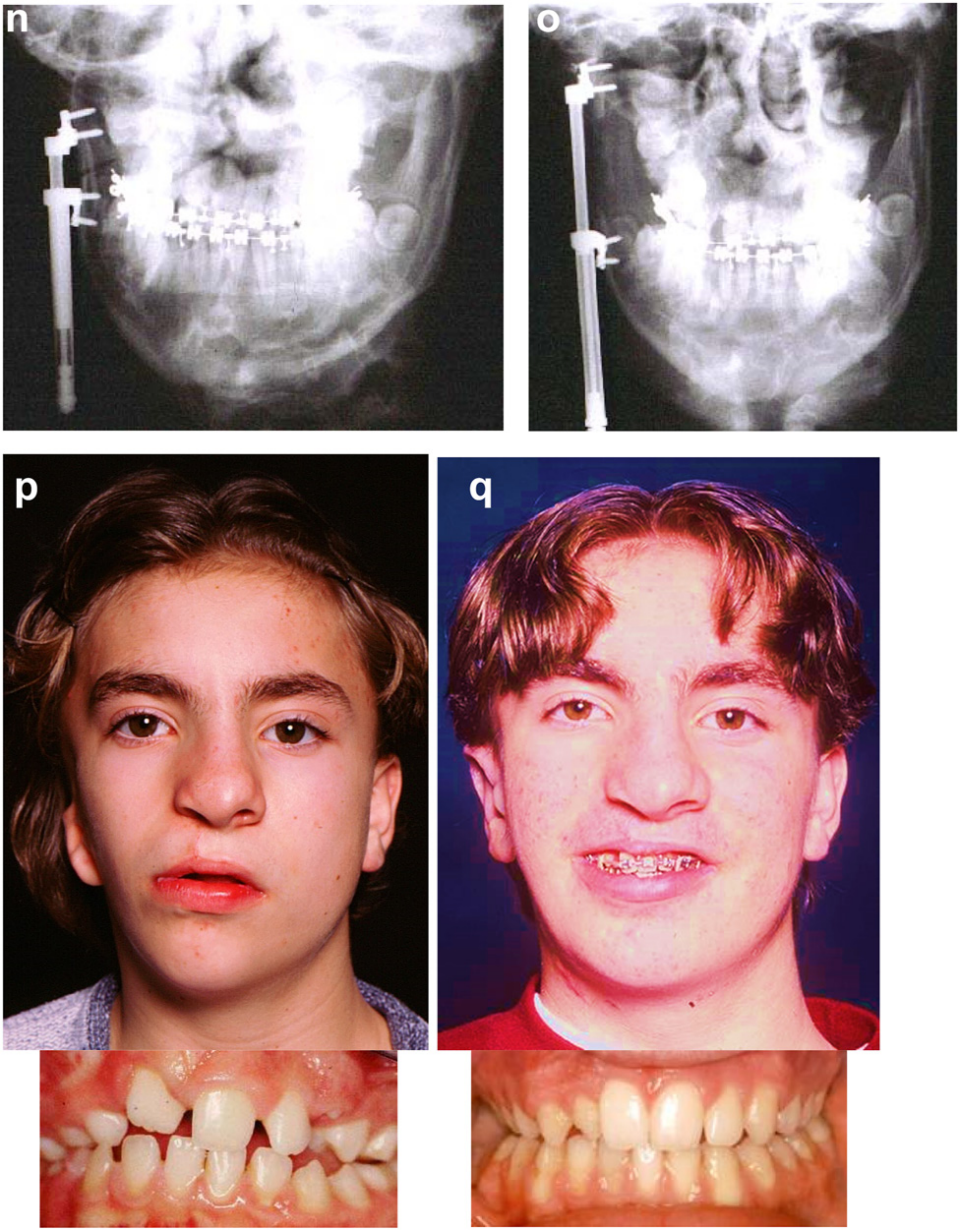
Planning for bimaxillary DO. (n and o) Radiographic outcome in case in Figure 12a and b – pre-distraction orthodontics has been carried out. (p and q) Before and after orthodontics and bimaxillary distraction.
